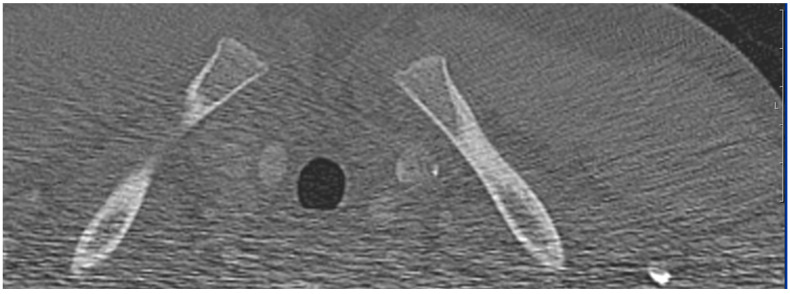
CT image of a 14-year-old subject with the right and left clavicle.
